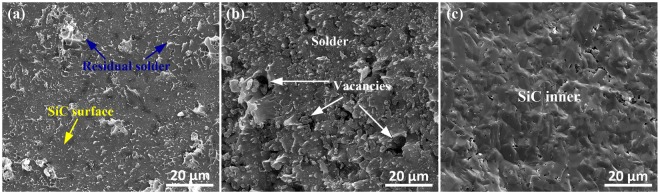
Secondary electrons SEM images of fracture surfaces of SiC joints. (a) At the SiC side with ultrasonic action for 0.1 s; (b) at the solder seam side with ultrasonic action for 0.1 s; (c) at the SiC side with ultrasonic action for 1 s.
